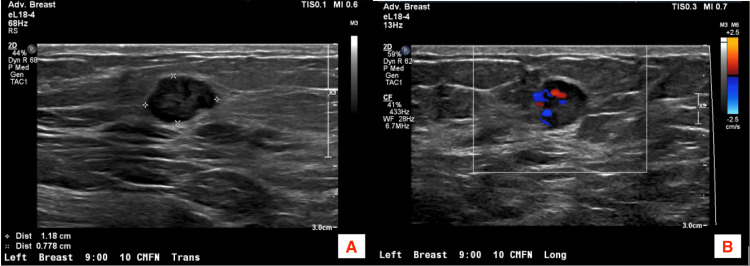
Ultrasound confirms the findings of the mammogram. (A) An ovoid circumscribed hypoechoic mass measuring 1.2 x 0.8 x 0.8 cm at the 9:00 position located 10 centimeters from the nipple (CFN). (B) There is internal vascular flow.
